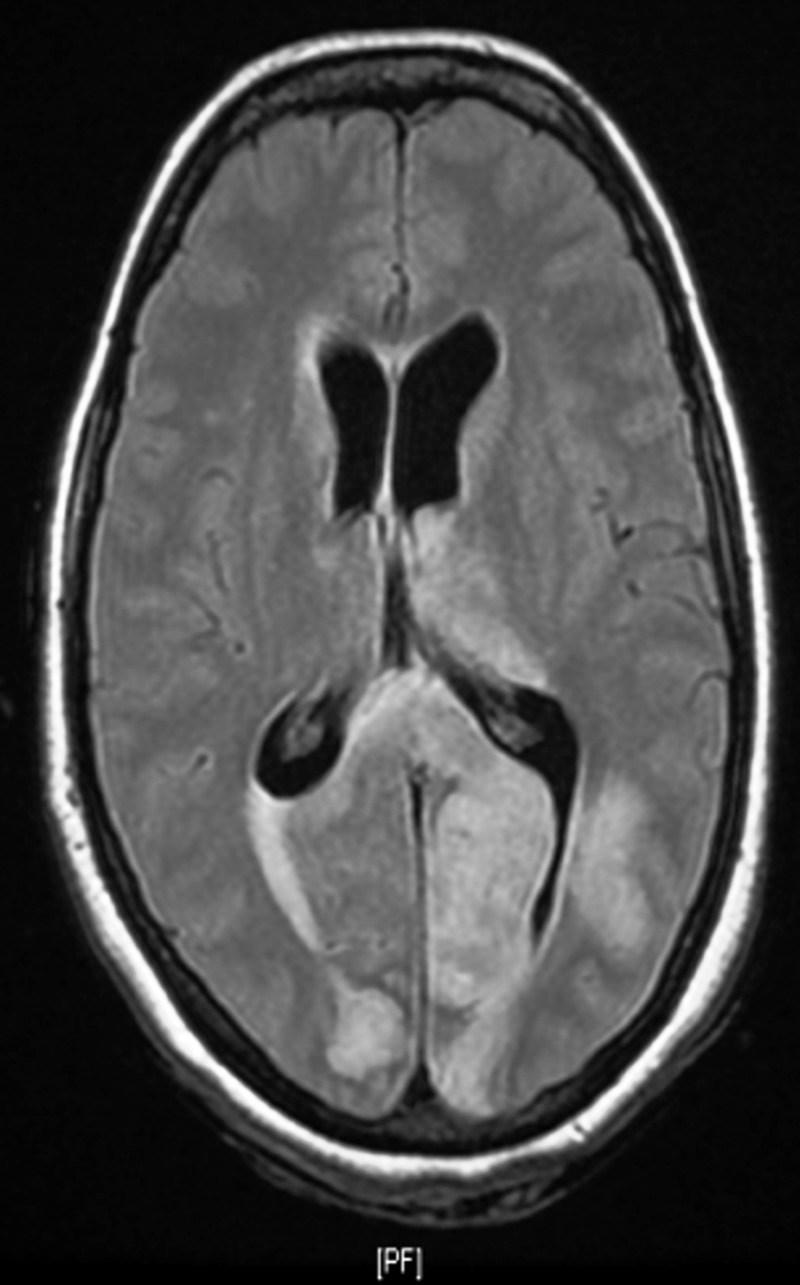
Fluid-attenuated inversion recovery (FLAIR) image of brain magnetic resonance imaging (MRI) showing hyperdensities in the cortex and subcortical white matter of the left parietooccipital lobe, right occipital consistent with posterior reversible leukoencephalopathy syndrome
